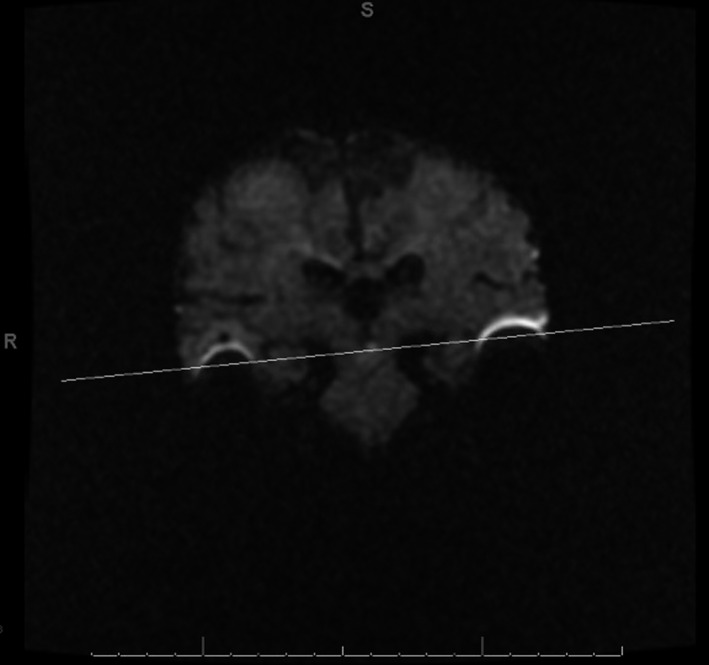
Coronal DWI with the level of the axial image in Figure 3 annotated with a white line
